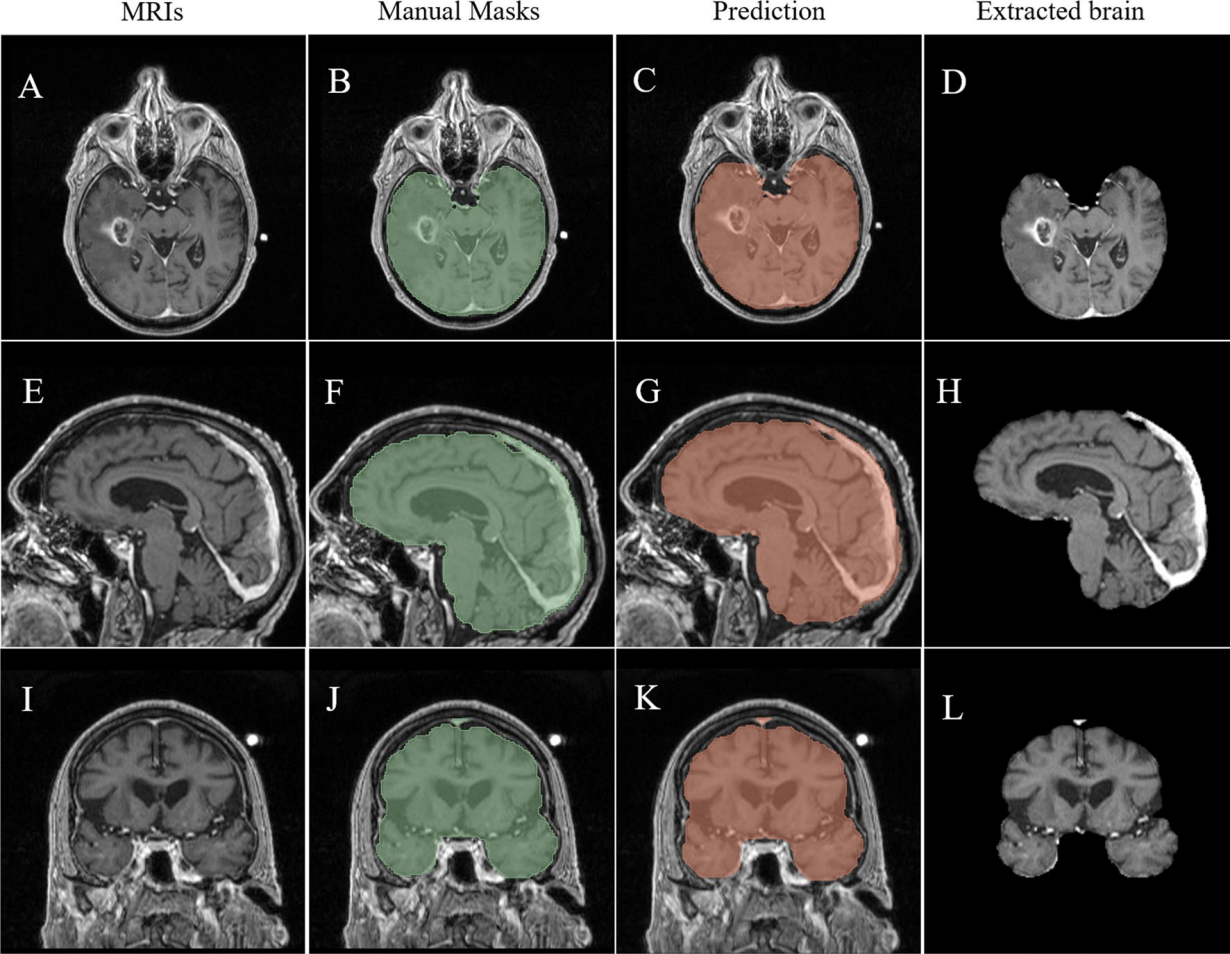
A brain extraction example for temporal glioblastoma from external test. A–D Axial views. E–H Sagittal views. I–L Coronal views. Segmentation performance of this case is DSC of 0.984, HD of 7.681 mm. MRIs, magnetic resonance imagings
